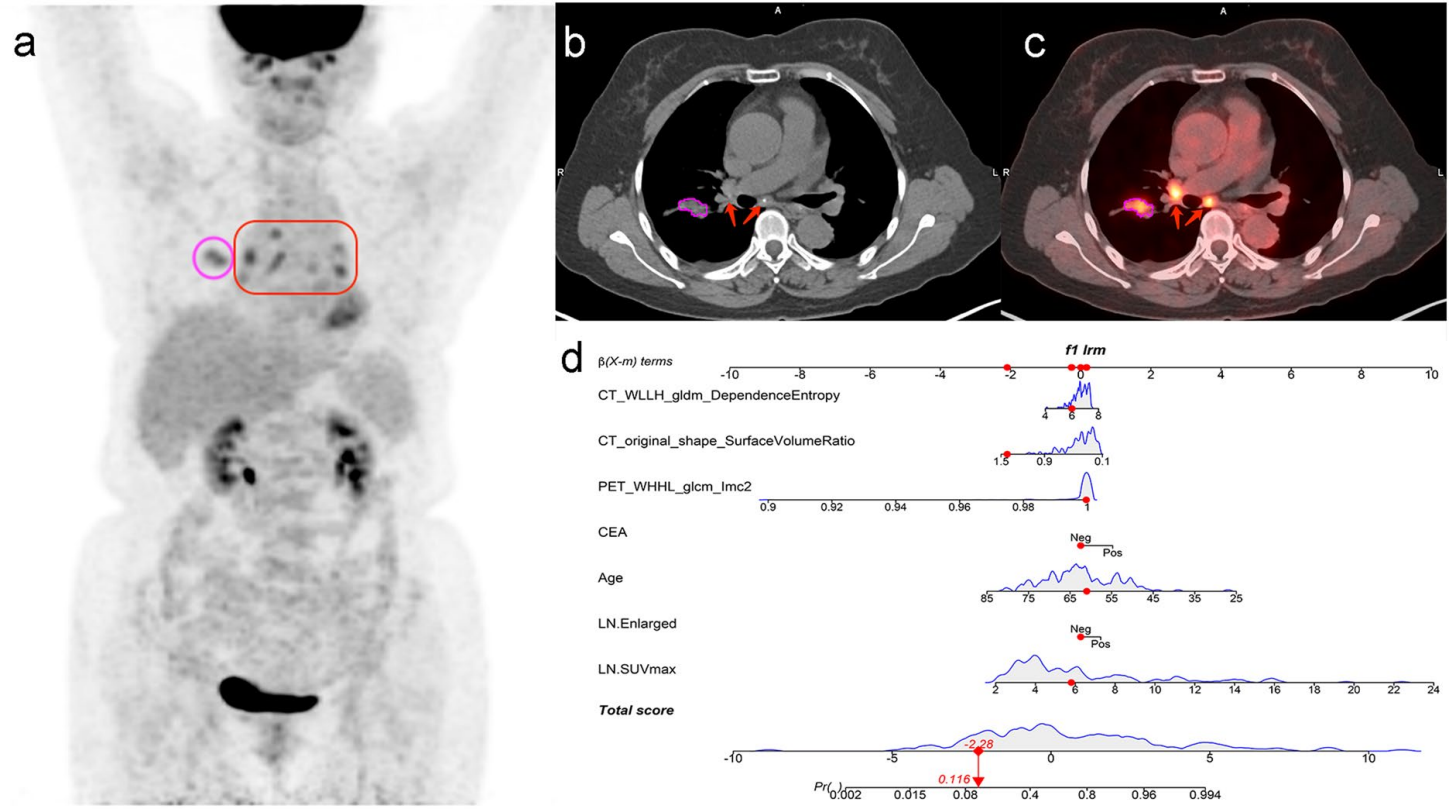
Example of nomogram clinical use. The preoperative whole-body PET/CT of this 61-year-old female with negative CEA status indicated the primary tumor was located in the right upper lobe (purple circle), with hypermetabolic mediastinal–hilar LNs (red square and arrows), and without distant metastasis (a–c). After completing the radiomics process (b, c) and applying the nomogram (d), the LNM probability of this patient was 0.12 (< 0.55), indicating a low risk of LNM. The pathological result of lobectomy with SND confirmed the negative status of mediastinal–hilar LN (0/7). The nomogram could improve the accuracy of hypermetabolic mediastinal–hilar LNs evaluation in lung cancer
